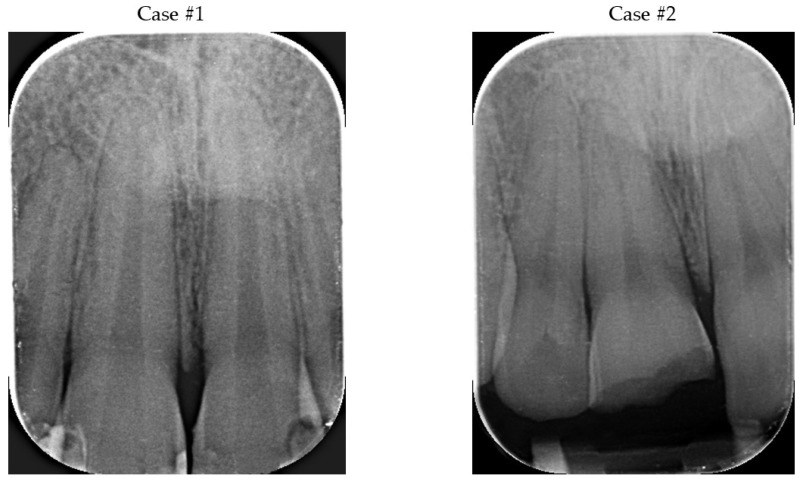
The preoperative X-rays.
